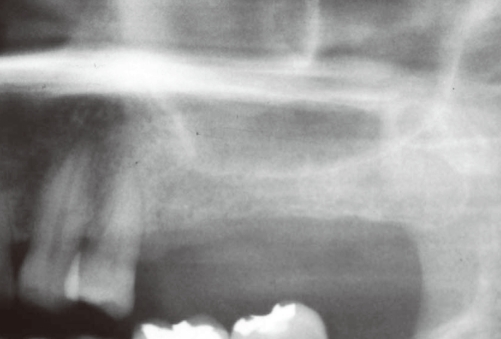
Pneumatization on the left maxillary sinus was observed in the preoperative panoramic radiograph.
